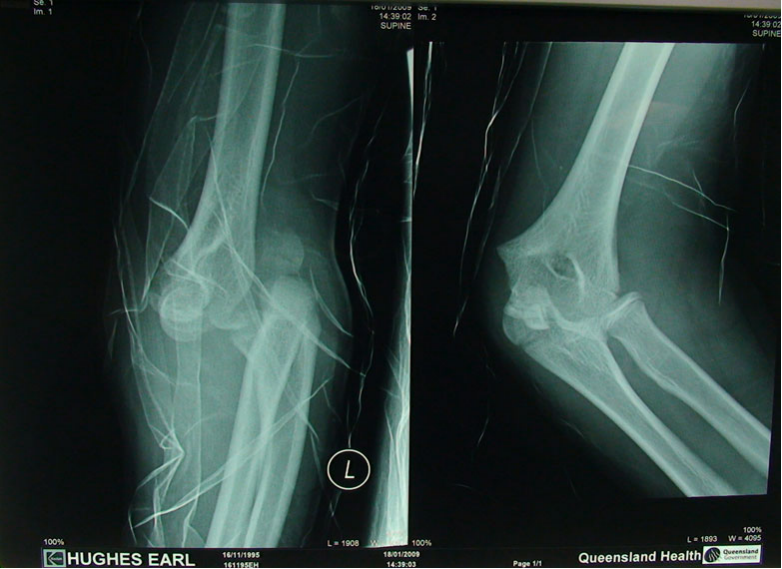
Injury X-ray 1 (showing dislocated elbow with biepicondylar fractures).
